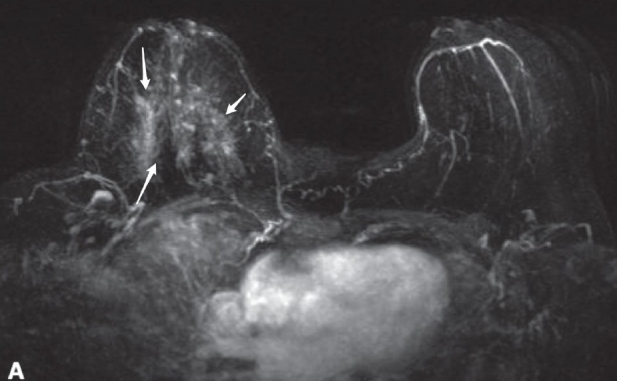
Pre-operative breast MRI in a 62-year-old woman with biopsy-proven high-grade ductal carcinoma *in situ* demonstrates diffuse non-mass-like enhancement (arrows) in the right breast, classified as BI-RADS 6Kinetic techniques analyze dynamic lesion enhancement and generate signal intensity/time curves. The shapes of these curves offer a wealth of information to help differentiate between benign and malignant lesionsThe initial enhancement phase is analyzed within the first 1–2 min after injection. The descriptors are slow, medium, or rapidThe delayed phase refers to the enhancement pattern after 2 min or after the curve starts to change. This curve is described as persistent, washout, or plateau. Persistent refers to continued increase in signal intensity over time. Washout describes a curve that shows decreasing signal intensity after peak enhancement. A plateau curve reaches the maximum signal intensity and then remains constant
